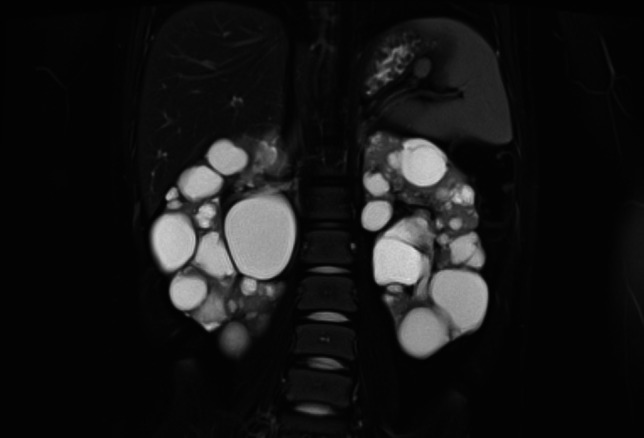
MRI images showing multiple bilateral renal cysts in an 8-year-old girl with *TSC2–PKD1* contiguous gene syndrome (MRI acquisition sequence: T2-weighted with fat saturation)
